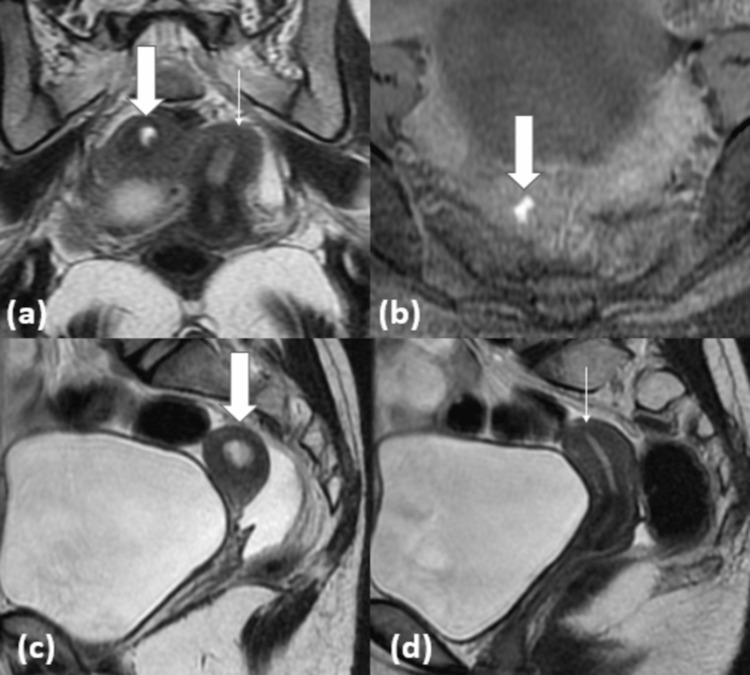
MRI images (a) coronal T2WI showing a non-communicating blood-filled rudimentary horn (block arrow) lying next to a mature unicornuate uterus (line arrow), (b) axial T1 fat-suppressed image showing hyperintense fluid in the horn on right side confirming hemorrhagic content in the obstructed rudimentary horn suggesting hematometra, (c) sagittal T2WI showing blood-filled rudimentary horn (block arrow) not communicating with the cervix, and (d) sagittal T2WI showing normal uterine horn (line arrow) communicating with the cervix.
